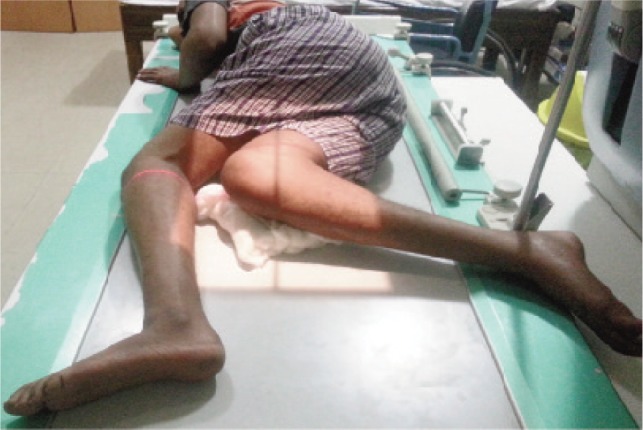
Patient postioning in stress radiography (active hamstring contraction test).
